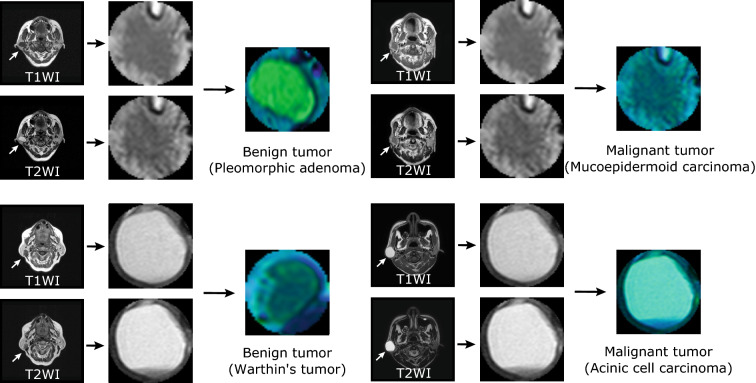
Examples of MR images of benign and malignant tumors. Original MR images (T1- and T2-weighted images) and their pseudo-color images of benign and malignant tumors.
